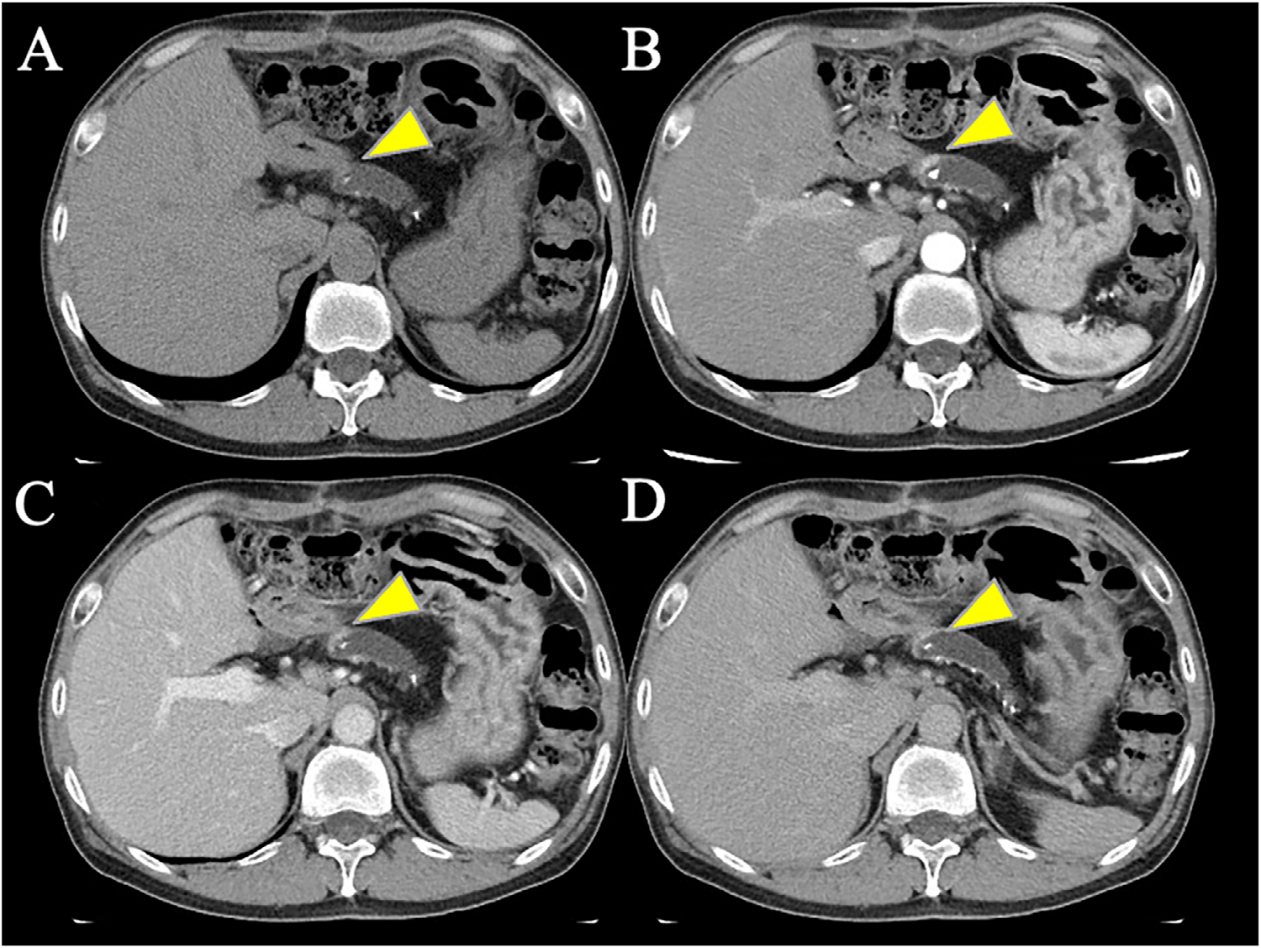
Findings on computed tomography (CT). (A) Plain. (B) Arterial phase. (C) Portal venous phase. (D) Delayed phase. On contrast‐enhanced CT, a slight hypervascular mass was observed in the arterial phase at the location indicated by the arrow.
